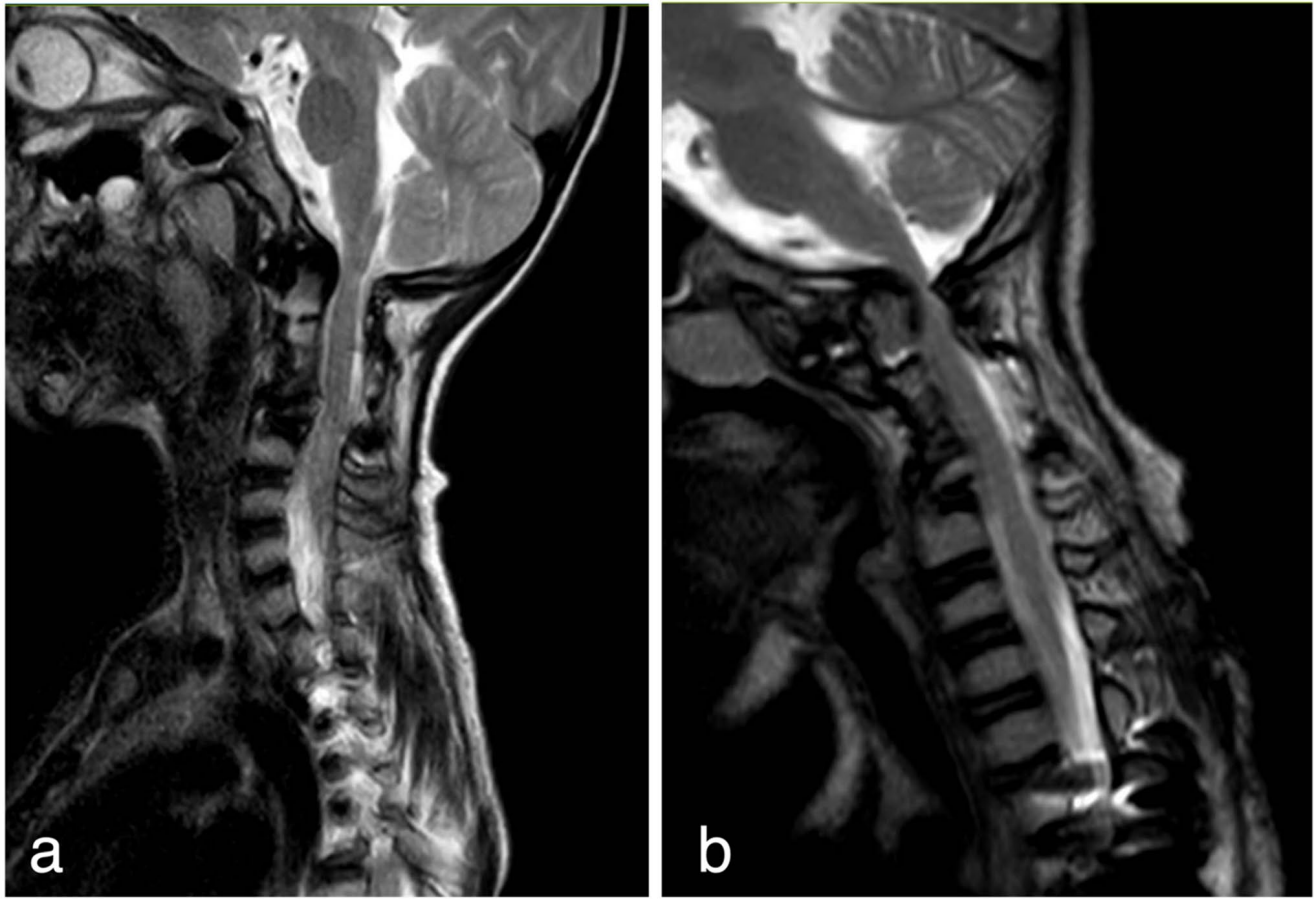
a Sagittal T2-weighted MRI at age 5 demonstrating initial mild odontoid protrusion through foramen magnum in a patient with Ehlers-Danlos syndrome. b Follow-up sagittal T2-weighted MRI at age 7 showing progression to severe basilar invagination with brainstem impingement, necessitating urgent surgical decompression and fusion
